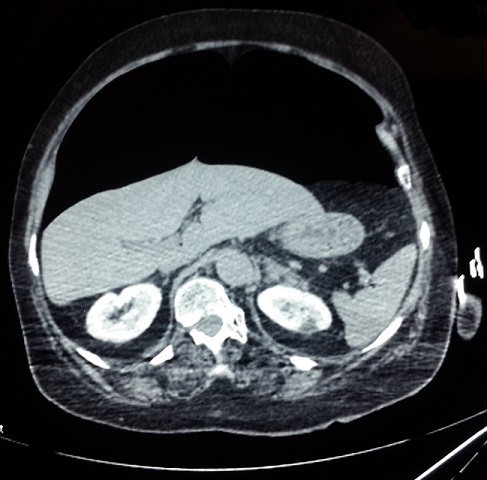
Thoraco-abdominal computed tomography scan performed one hour after the occurrence of the abdominal distension. It revealed a massive pneumoperitoneum, without intraperitoneal effusion, pneumothorax or pneumomediastinum
